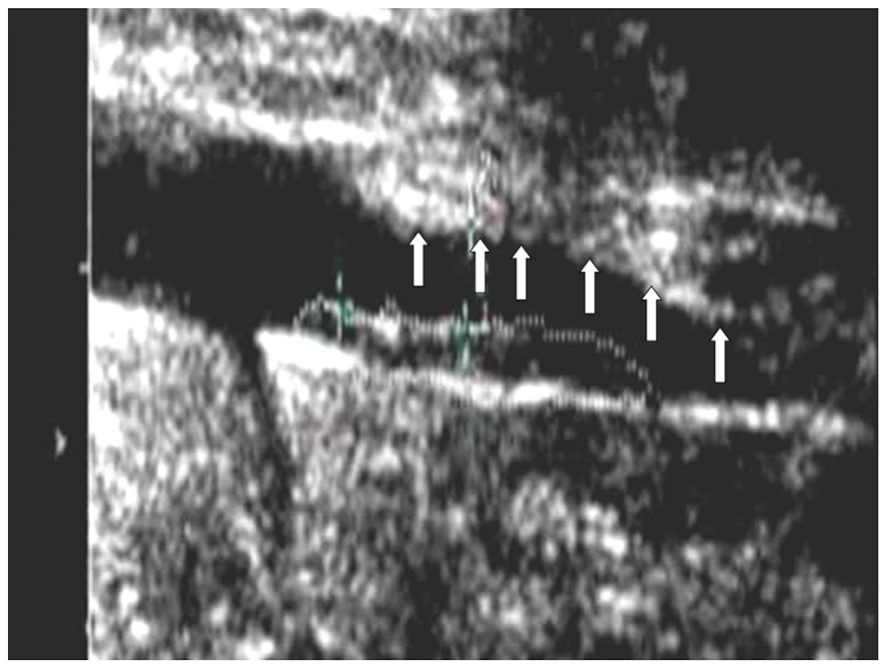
Rabbit abdominal aorta atherosclerotic plaque on the near aortic wall (arrows) was displayed clearly by ultrasonography.
